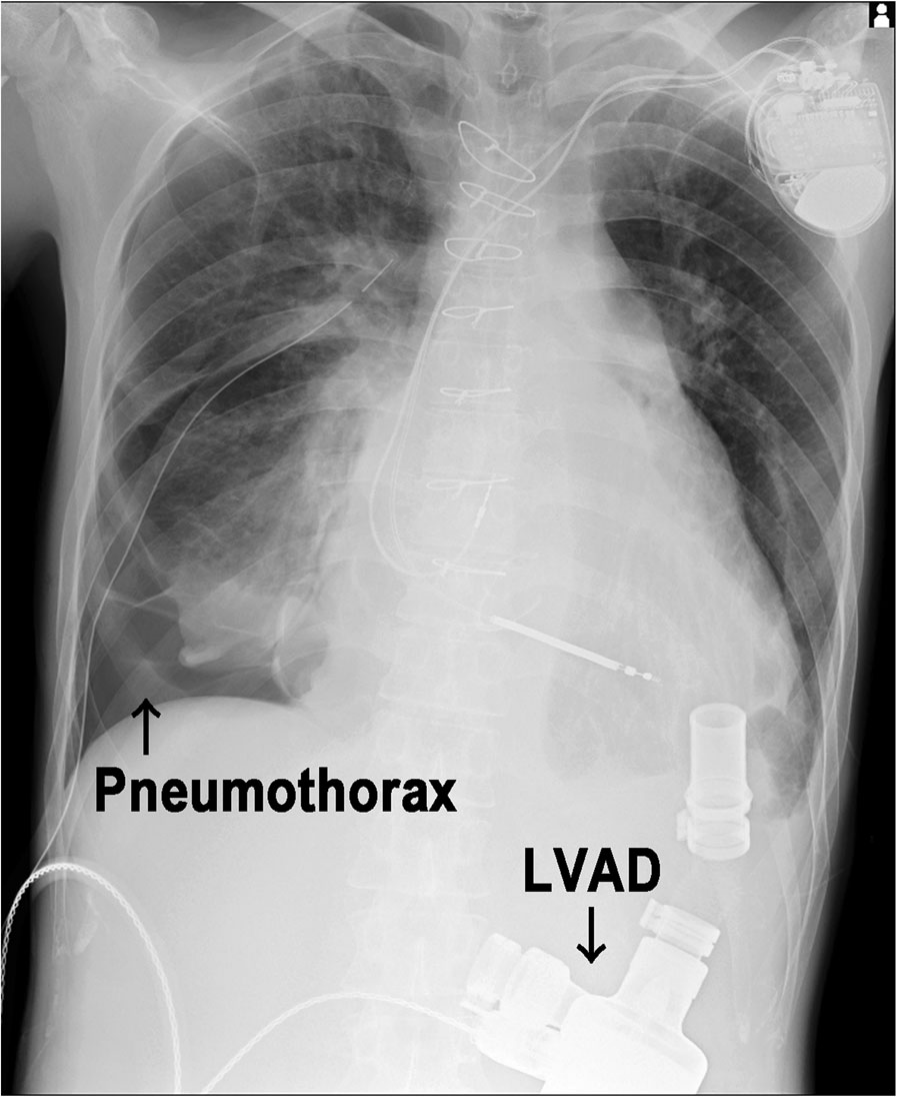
Chest radiograph. Chest radiograph showing the right-side pneumothorax with a chest tube. LVAD: left ventricular assist device
